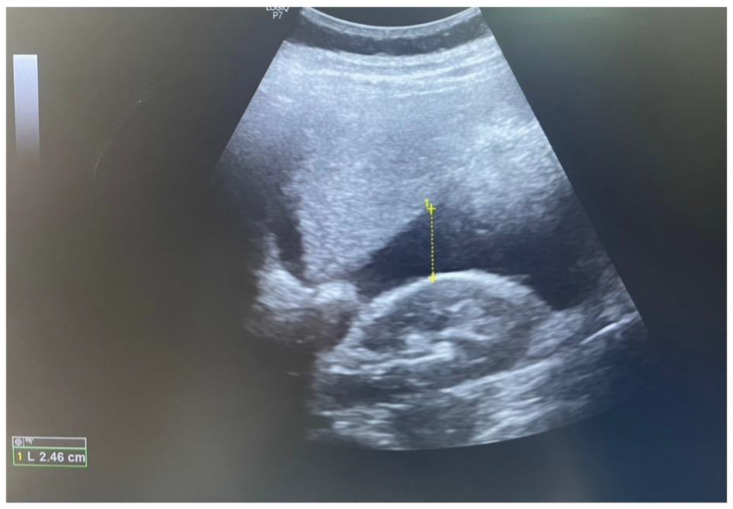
Abdominal ultrasound scan (yellow dotted line indicated the presence of fluid in the hepato-renal space).
